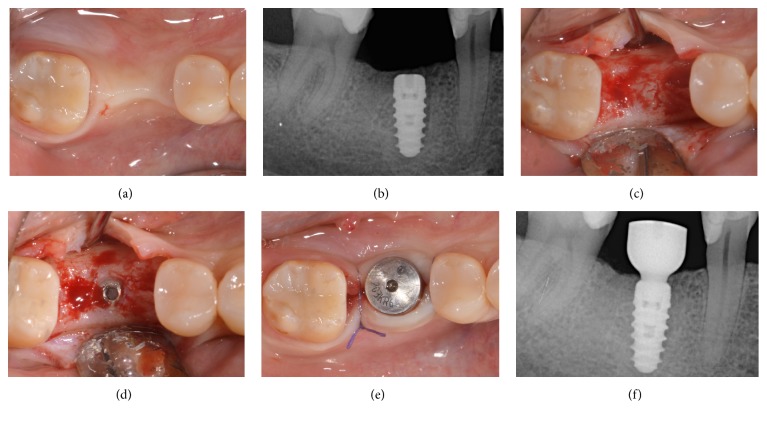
Immediate implant placement of a mandibular first molar (#46). Soft tissue healing 3 months after implant placement (a); periapical radiograph after 3 months of submerged healing (b); second-stage surgery and the alveolar ridge 3 months after implant placement (c); fixture exposure during second-stage surgery (d); placement of the healing abutment and sutures (e); periapical radiograph after insertion of the healing abutment (f).
